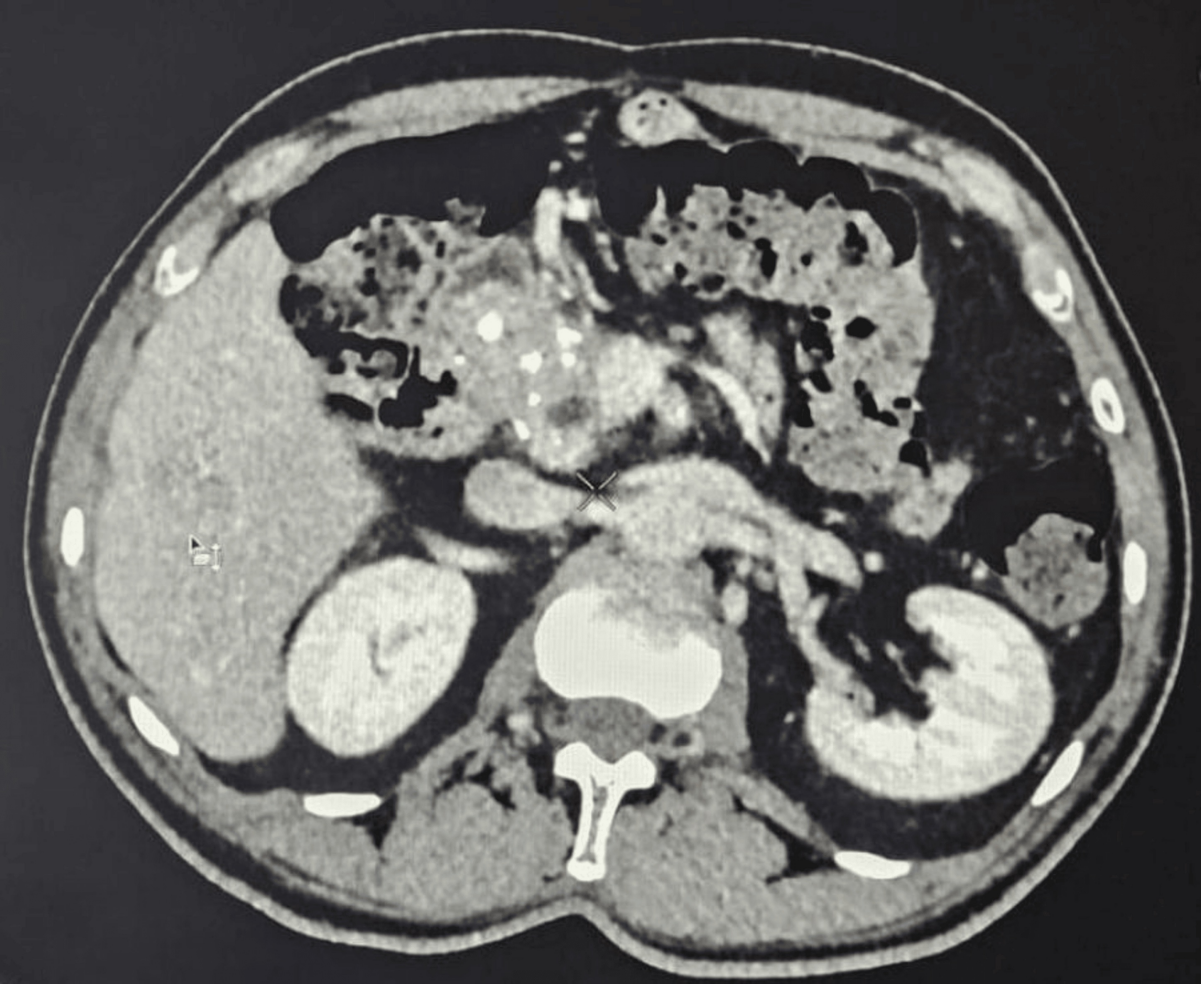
Contrast-enhanced CT showed the hepatic lesion with marked reduction in size and density appearing as subtle irregular faint hypodensity
